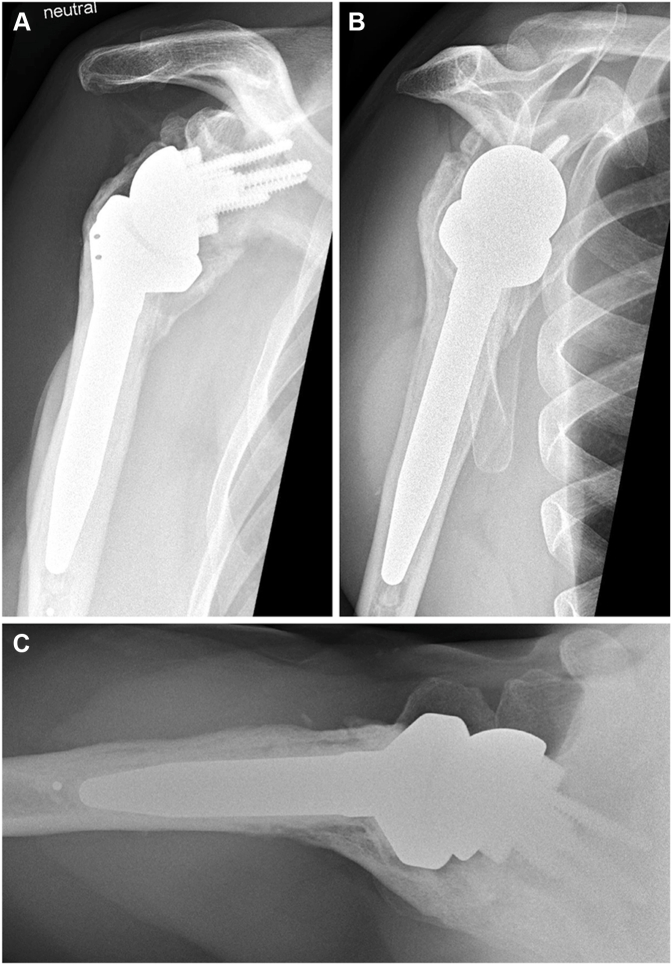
(A) Anteroposterior, (B) Neer, and (C) axial radiographs of the right shoulder at the 5-year follow-up showing complete glenohumeral ankylosis around the prosthesis.
